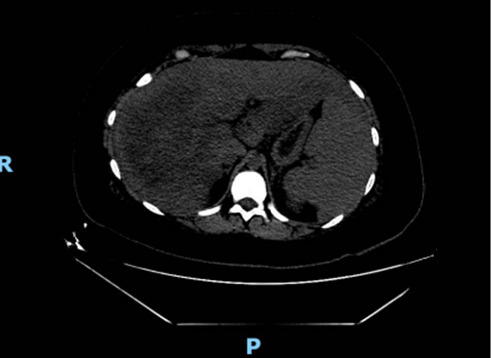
CT abdomen/pelvis with/without contrast demonstrating focal steatosis of the liver.
